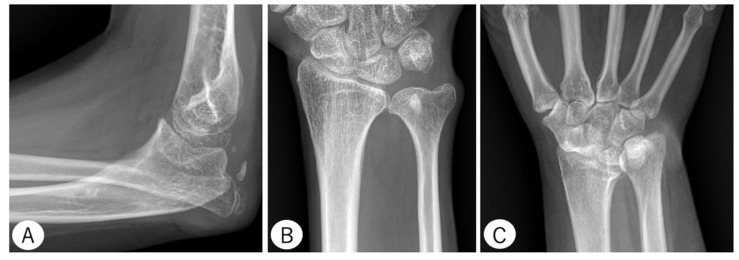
Radiographs of example cases. Patient 3 at age 11 years shows an angularly deformed proximal ulna and posteriorly dislocated radial head (A). Patient 12 at age 39 years shows a narrow ulnar diaphysis and an enlarged distal area (B). Patient 11 at age 31 years shows a fused scaphoid and trapezium (C).
